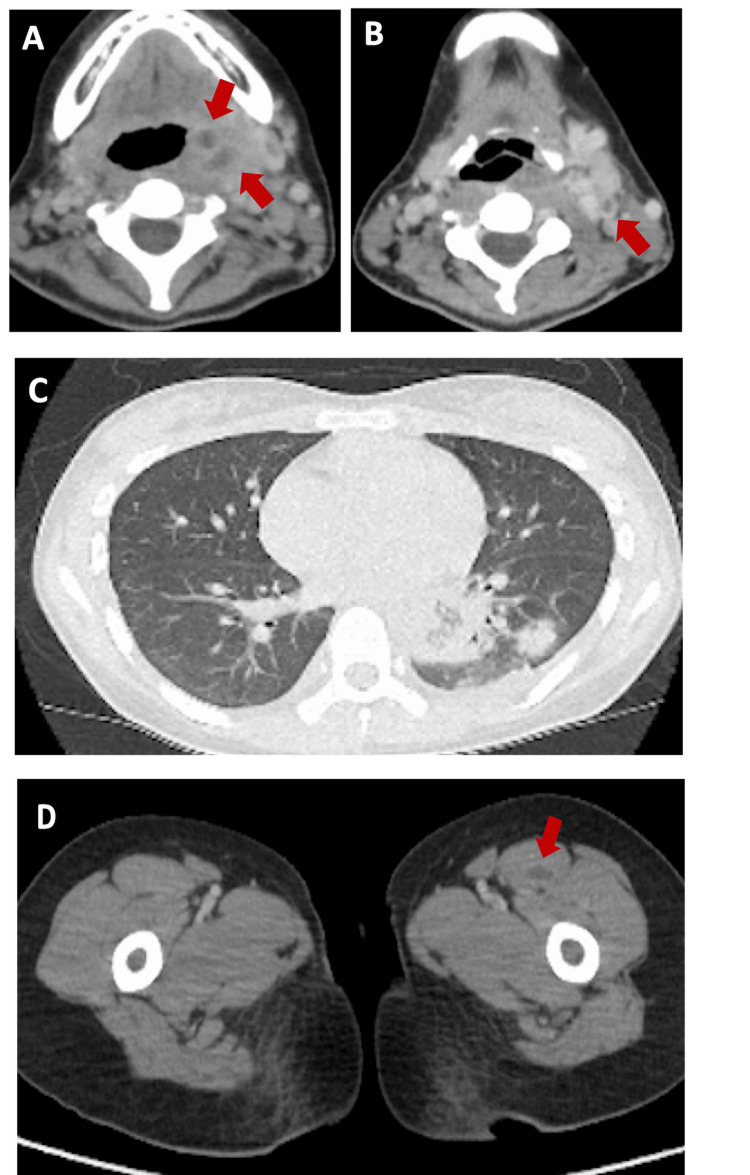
Imaging study A. CT of the neck with contrast shows that two low-density areas with ring enhancement are observed in the left pharyngeal wall, suspected to be abscesses (red arrows: both are approximately 4 mm x 4 mm x 5 mm).
